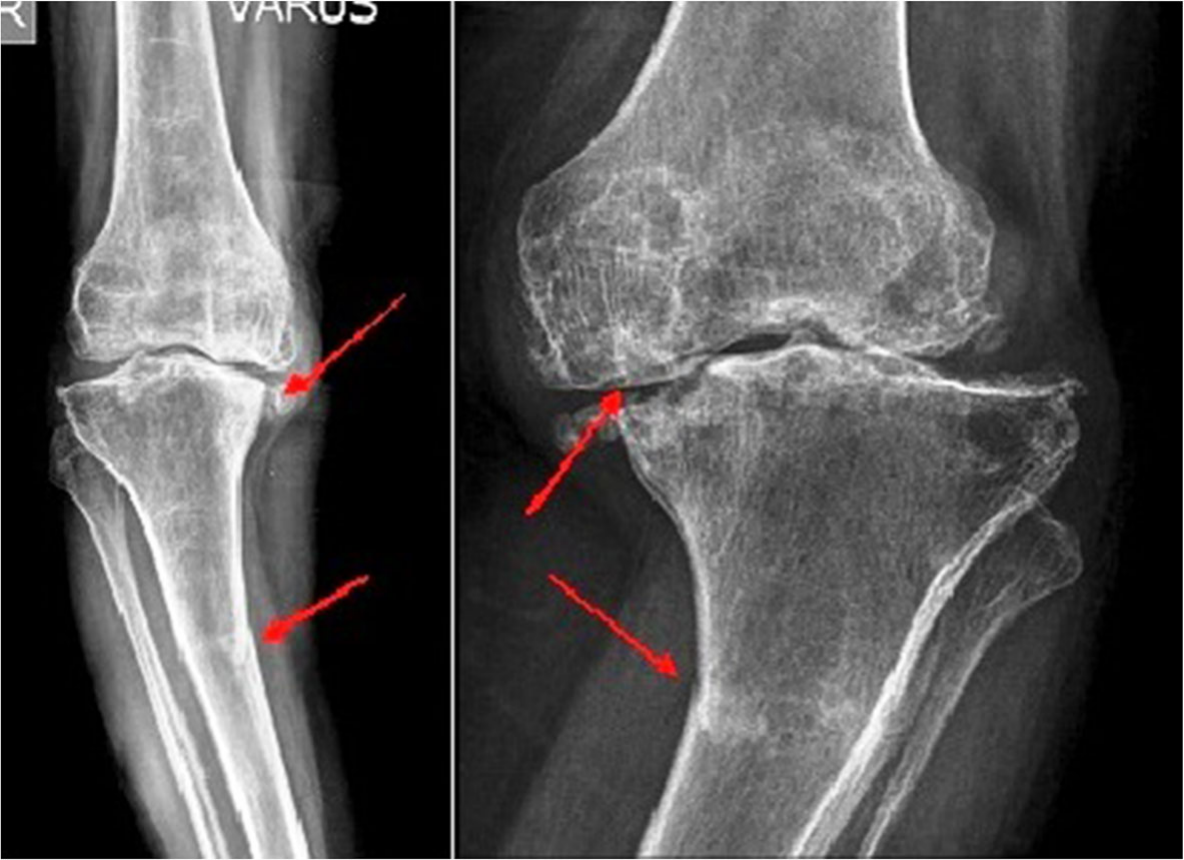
An anteroposterior X-ray of the knee showing reduction degenerative changes, irregularity, diminution of medial joint space, osteophytes, varus deformity (arrow showing features suggestive of osteoarthritis) with stress fracture of proximal tibia (arrow showing stress fracture)
